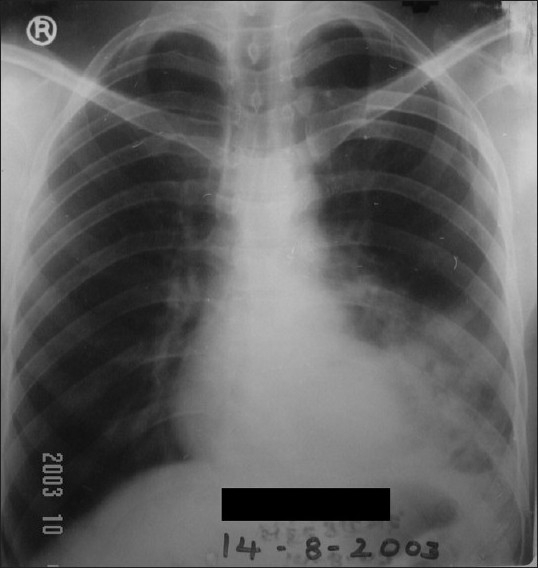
Chest X-ray revealing double-contour of the left cardiac border and inhomogeneous infiltrates on the left lower zone
